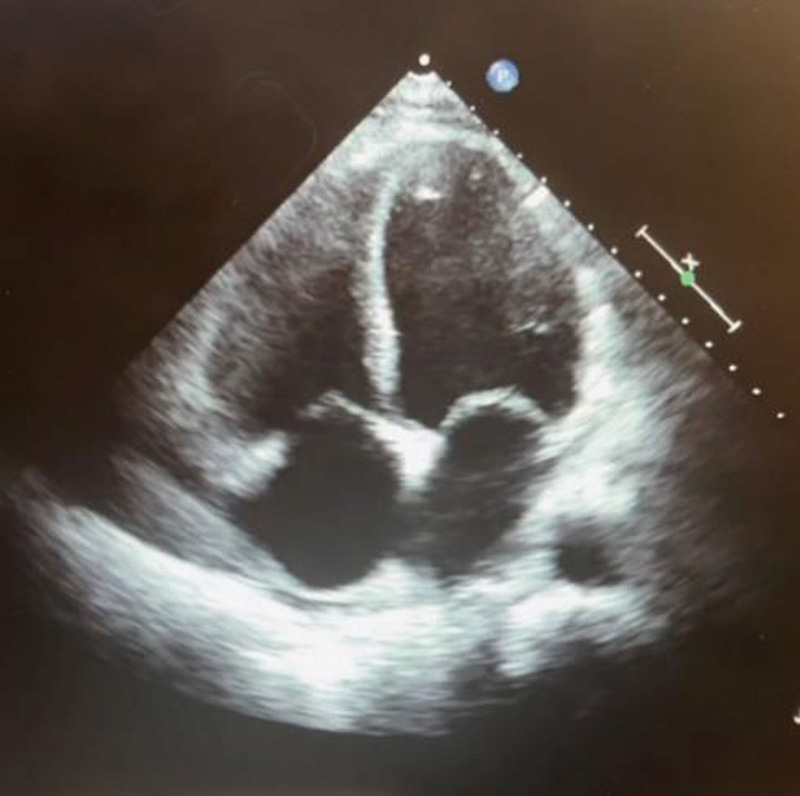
A TTE image showing the 4-chambers with no abnormalities within the RA. RA = right atrium, TTE = transthoracic echocardiography.
